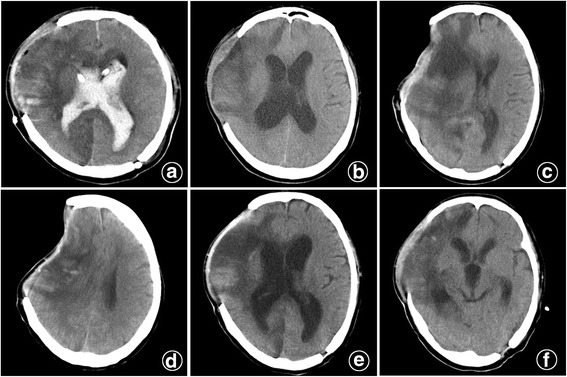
a A CT after the surgery of subdural hematoma in the frontal temporal lobe cleaning, craniectomy and ventricular drainage. b CT showed a hydrocephalus three-week later after the surgery. c, d The patient had a SSFS and midline shift after the lumbar puncture. e, f After placing the patient in a Trendelenburg positon and giving intravenous fluid, SSFS and midline shift disappeared one day later
